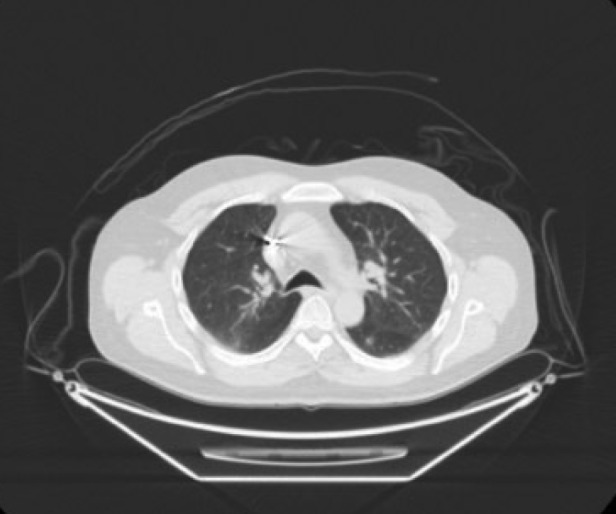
August 2012 CT scan shows excellent treatment response after 7 cycles of capecitabine/oxaliplatin/cetuximab.
